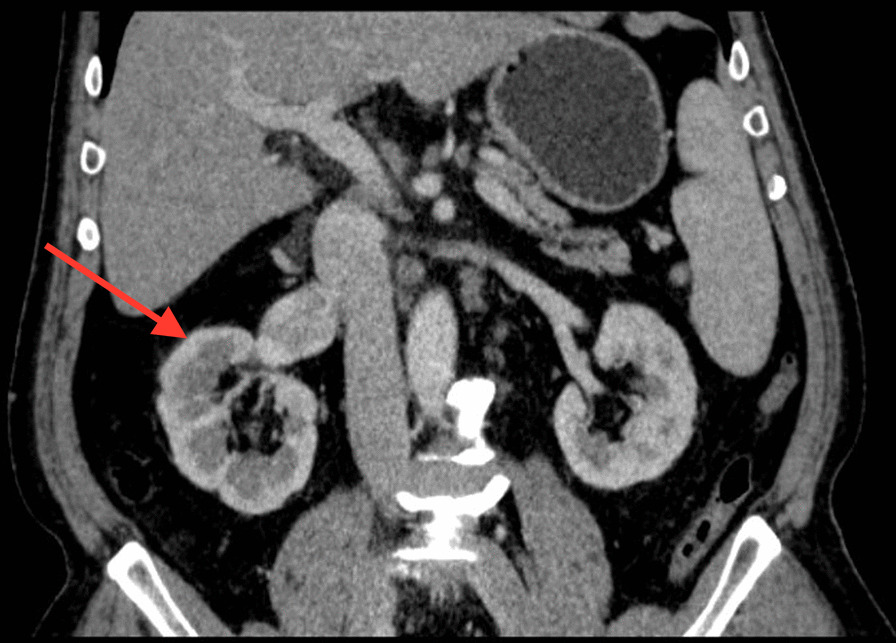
CT showed a mass in the right kidney. The right kidney was significantly enlarged, and the right renal vein was significantly thickened
